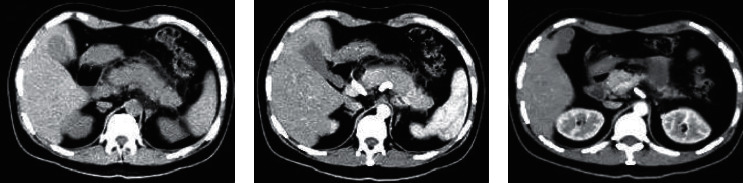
CT images of acute pancreatitis (patient with acute simple pancreatitis, female, 57 years old).
